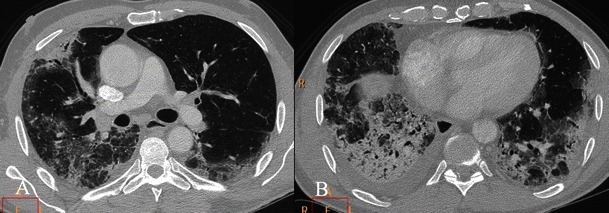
AIP pattern in a 56-year-old man. The patient was admitted to our emergency room for severe dyspnoea (leukocytes = 12,000/mmc; neutrophils 90 %; D-dimer 452 ng/ml; CRP 7,10 mg/dl; ERS 78 mm/h). CT images show mild pleural effusion, ground-glass opacifications and inhomogeneous consolidations. Differential diagnosis includes several diseases, such as acute exacerbations of IIPs, NSIP, COP, infectious diseases and collagen-related lung fibrosis
